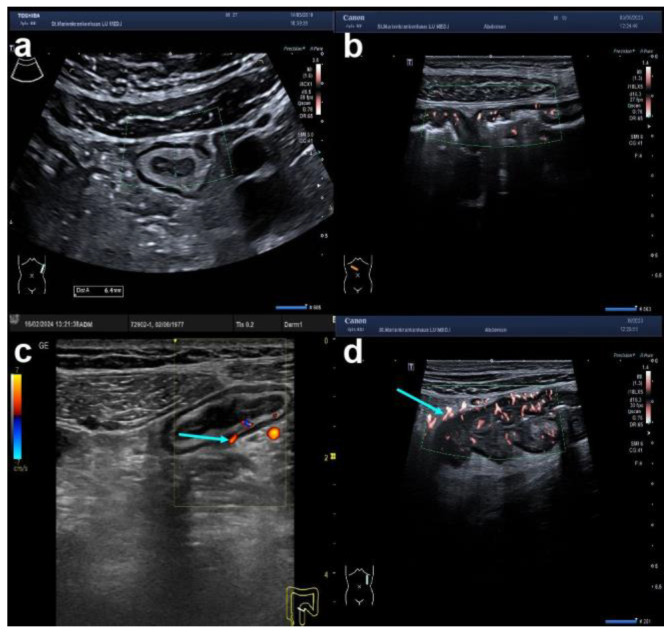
Variants of bowel wall vascularisation in IBD using colour Doppler signalling of colonic walls. Shown are typical examples employing the modified Limberg classification: (a) transverse section of the descending colon showing preserved layers with increased BWT but no signals on CDS employing superb microvascular imaging (SMI) (Limberg 1); (b) longitudinal section of the right transverse colon with orange dots within the blurred intestinal wall using SMI (Limberg 2); (c) oblique section of the sigmoid colon with preserved BWS, increased BWT and stretches of blood vessels within the intestinal wall using colour Doppler signalling indicated by the turquoise arrow (Limberg 3); (d) longitudinal section of the descending colon with markedly increased vascularity in the intestinal wall extending into the pericolonic echoenhanced tissue (turquoise arrow) using SMI (Limberg 4).
